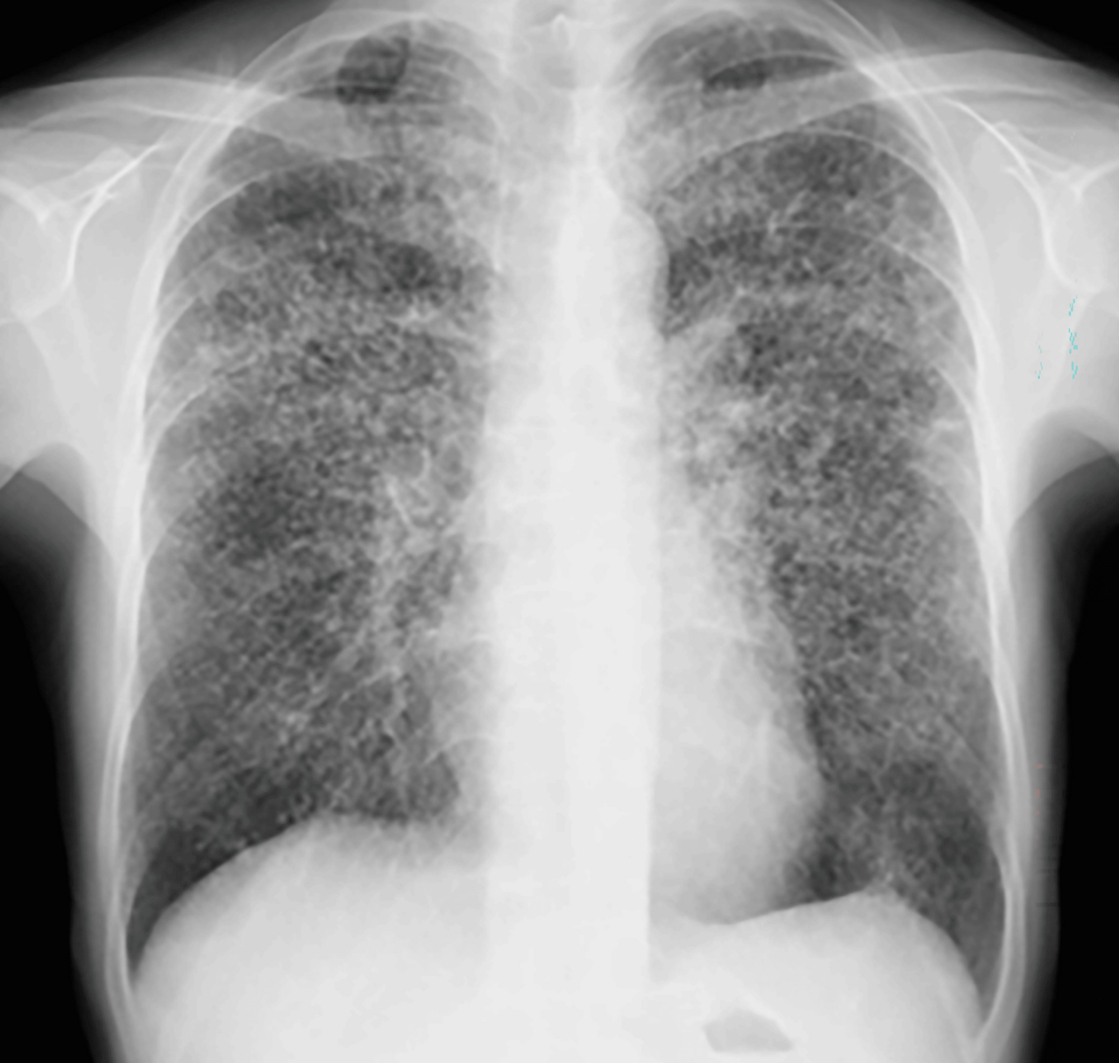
Chest radiograph (upright posteroanterior view) The chest radiograph demonstrates diffuse bilateral reticulonodular opacities throughout both lung fields.
